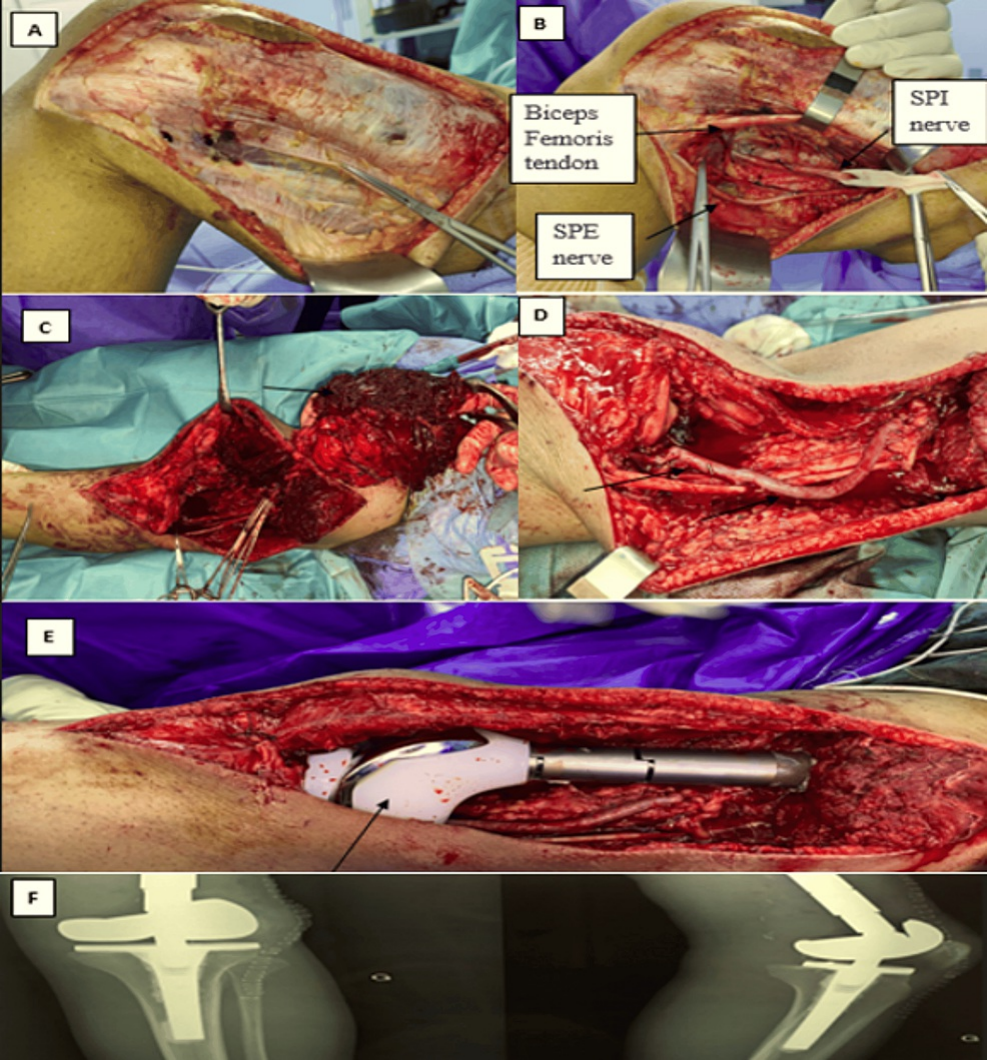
Intraoperative images of resection – reconstruction of the tumor. (A) Cutaneous and subcutaneous incision, centered on the tumor. (B) Neurolysis of the SPI and SPE nerves along with the lateral sural nerve. (C) Resection of the tumor. (D) Femoropopliteal bypass and ligation of the popliteal vein. (E) Placement of a definitive cemented massive knee prosthesis. (F) Postoperative x-rays.
